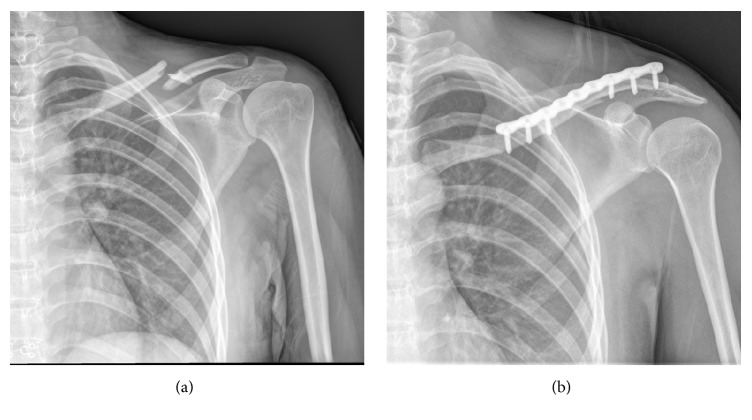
(a) Left closed comminuted midshaft clavicle fracture; (b) fracture end anatomical reduction could be seen in X-ray films after minimally invasive Kirchner wire temporary intramedullary fixation combined locking anatomical plate.
